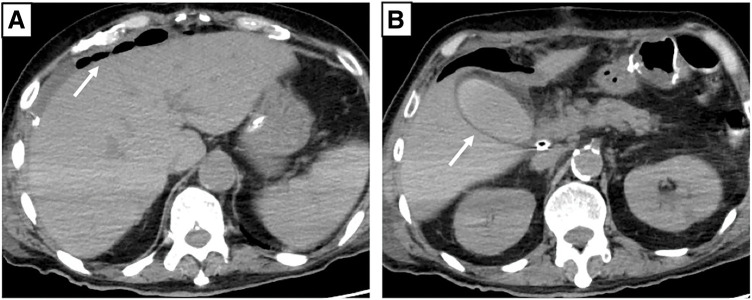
CT scan before reoperation. The CT scan shows no intrahepatic bile duct dilatation, and a white arrow indicates increased free air around the liver (A). A white arrow indicates thickening of the gallbladder wall (B). CT, computed tomography
